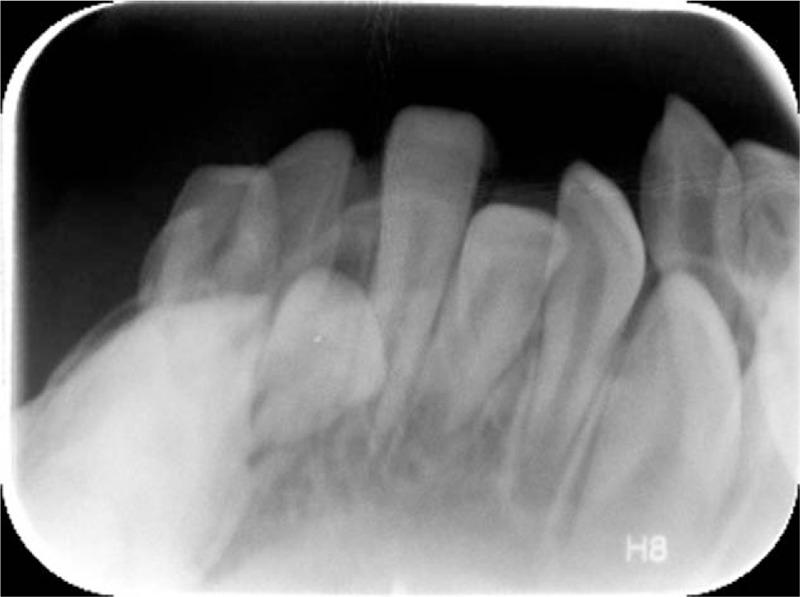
Mandibular occlusal radiograph from most recent appointment in 2018.
